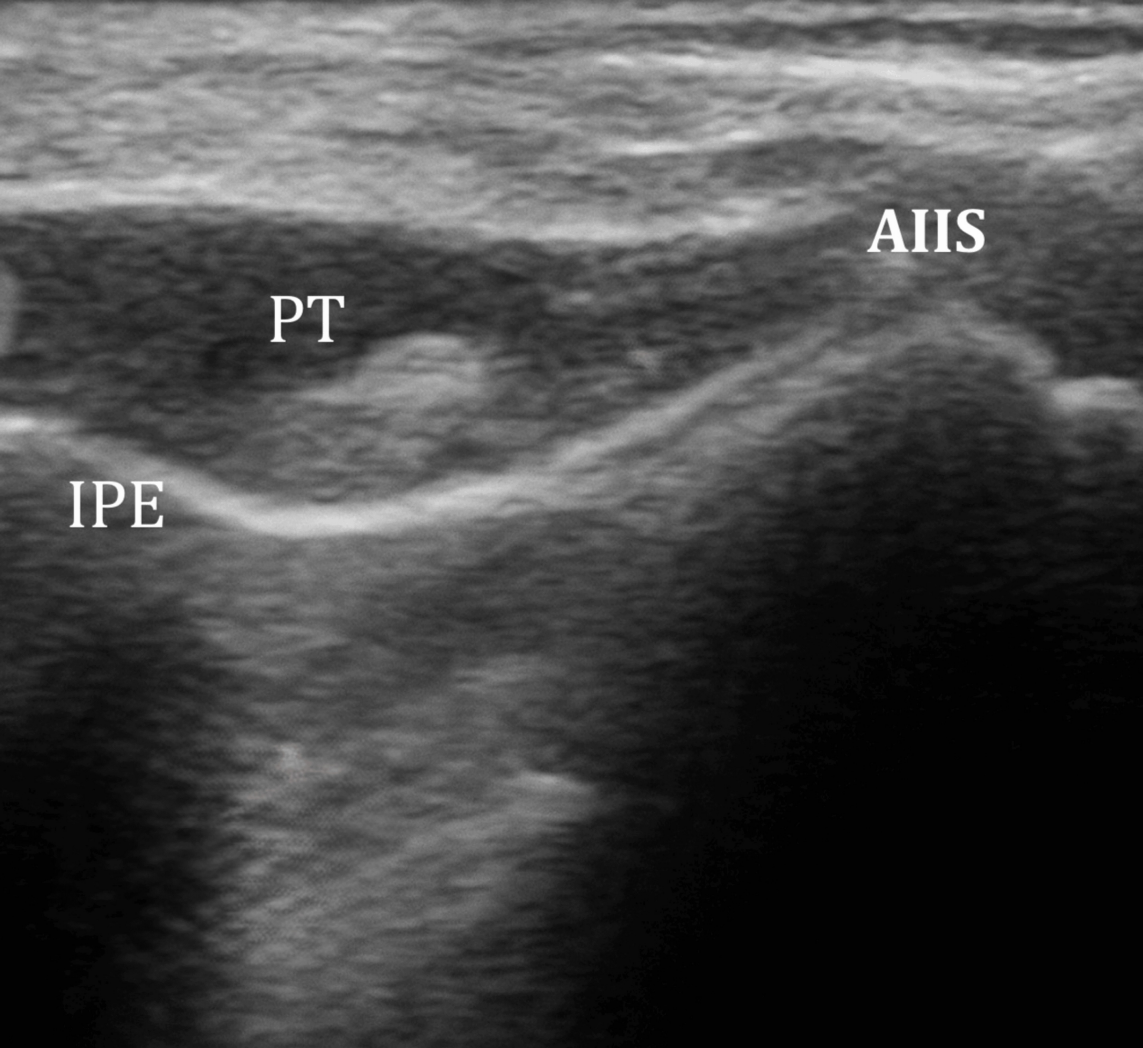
Ultrasound-guided PENG block landmarks and injectate deposition. The image displays the relationship between the anterior inferior iliac spine (AIIS), the psoas tendon (PT), and the iliopubic eminence (IPE). The local anesthetic is visualized as a hypo-echoic (dark) expansion in the fascial plane directly beneath the PT and superficial to the pubic ramus. FA, femoral artery; PENG, pericapsular nerve group
